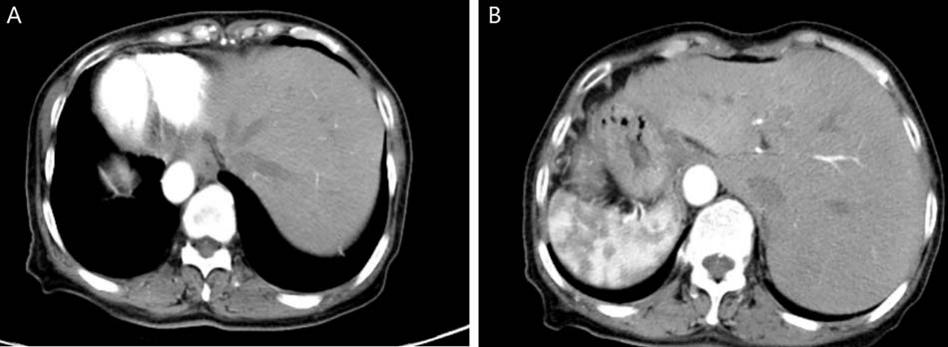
Enhanced CT of the upper abdomen showed that the heart was located on the right side of the thoracic cavity, the liver was located on the left side of the abdominal cavity (A), and the stomach and spleen were located on the right side of the abdominal cavity (B), confirming SIT.
